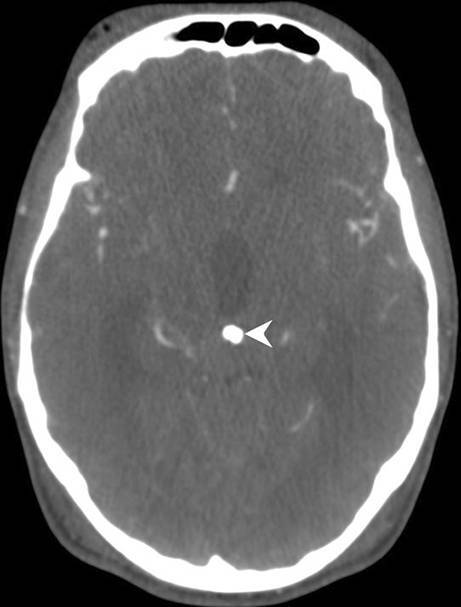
On axial postcontrast computed tomography (CT) images the lesion shows a firm, centrally located calcification (*arrowhead*)
